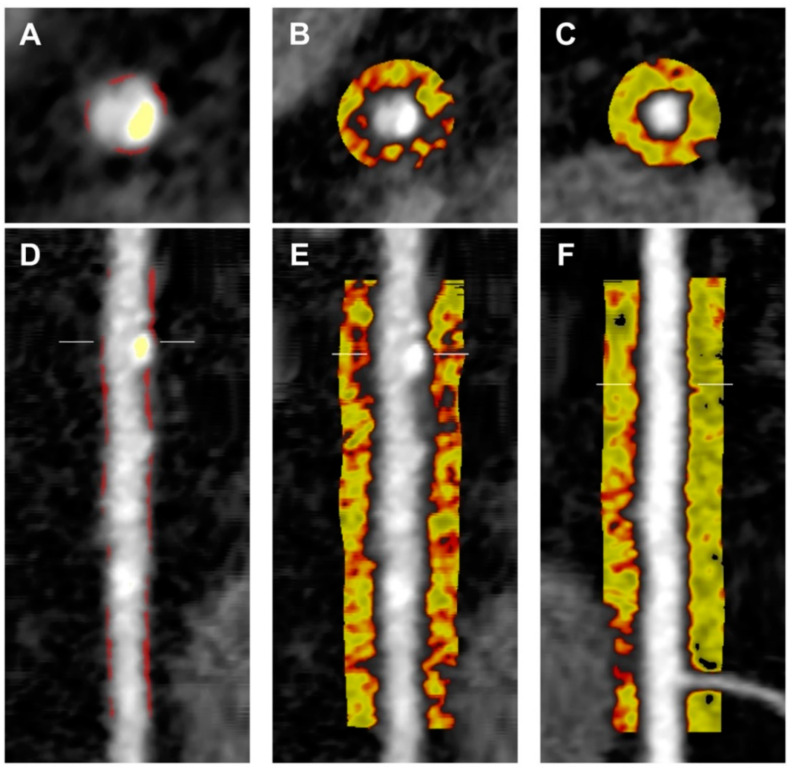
Case comparison of per-patient PCAT quantification. Comparison of the RCA of a patient with HRP versus a patient without HRP (control) as shown on coronary CTA. RCA in patient with HRP shown in cross-sectional (A) and longitudinal (D) views, with calcified plaque (yellow) and non-calcified plaque (red) highlighted. Representation of PCAT attenuation of patient with HRP (−75.6 HU) and control (−93.9 HU) shown in cross-sectional (B,C) and longitudinal (E,F) views. Colour map corresponds to CT attenuation in Hounsfield Units (HU) ranging from −190 HU (yellow) to −30 HU (red). Cross-sectional view in patient with HRP shown in panel (B) versus control shown in panel (C). Longitudinal view in patient with HRP shown in panel (E) versus control shown in panel (F). RCA: right coronary artery.
